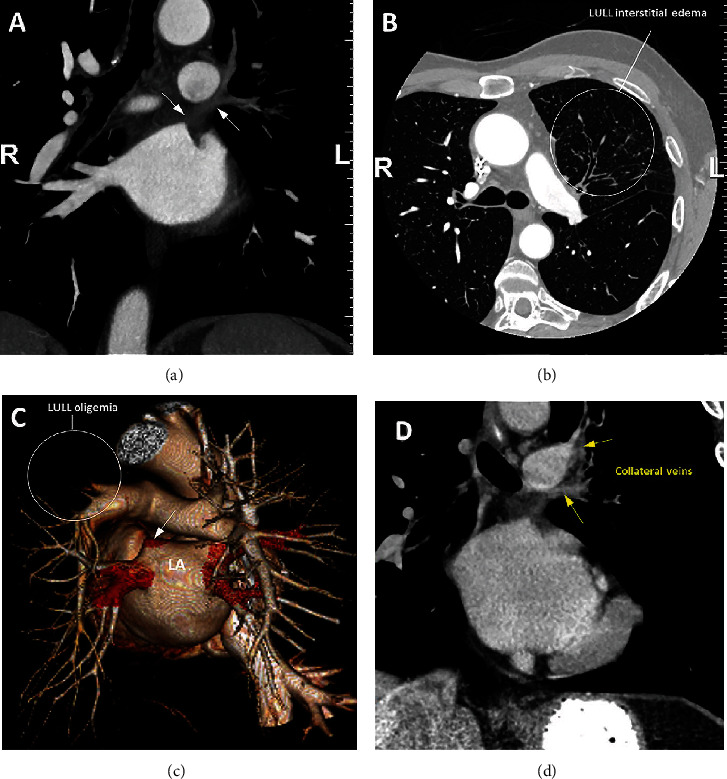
Coronal oblique view (a), axial view (b), and volume-rendered 3D reconstruction (c) from an arterial phase CT angiogram demonstrate absence or stump of the left superior pulmonary vein (white arrows) and left upper lobe oligemia ((c), circled) and chronic interstitial edema ((b), circled). Coronal oblique vascular reconstruction from a delayed venous phase CT angiogram (d) demonstrates small collateral draining veins (yellow arrows). LULL = left upper lung lobe.
